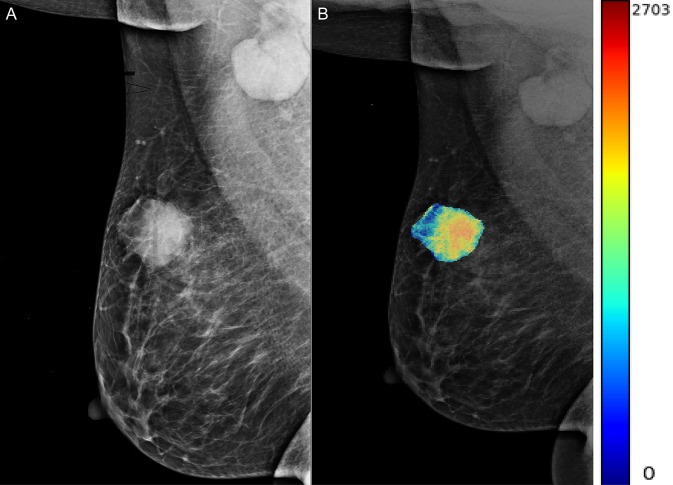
The woman, 56 years old, had triple-negative breast cancer, indicating a high tumor-infiltrating lymphocyte level in her right breast (arrow). (A) Right mediolateral oblique X-ray shows an uneven and smooth mass in the right breast. (B) X-ray image of mass density color overlay, showing uneven and smooth mass.
